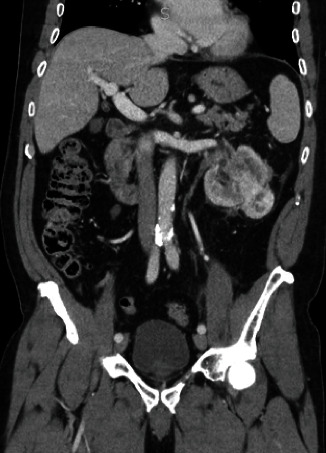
Computed tomography of renal mass demonstrating exophytic and infiltrative components of a 9 cm left interpole renal mass.
